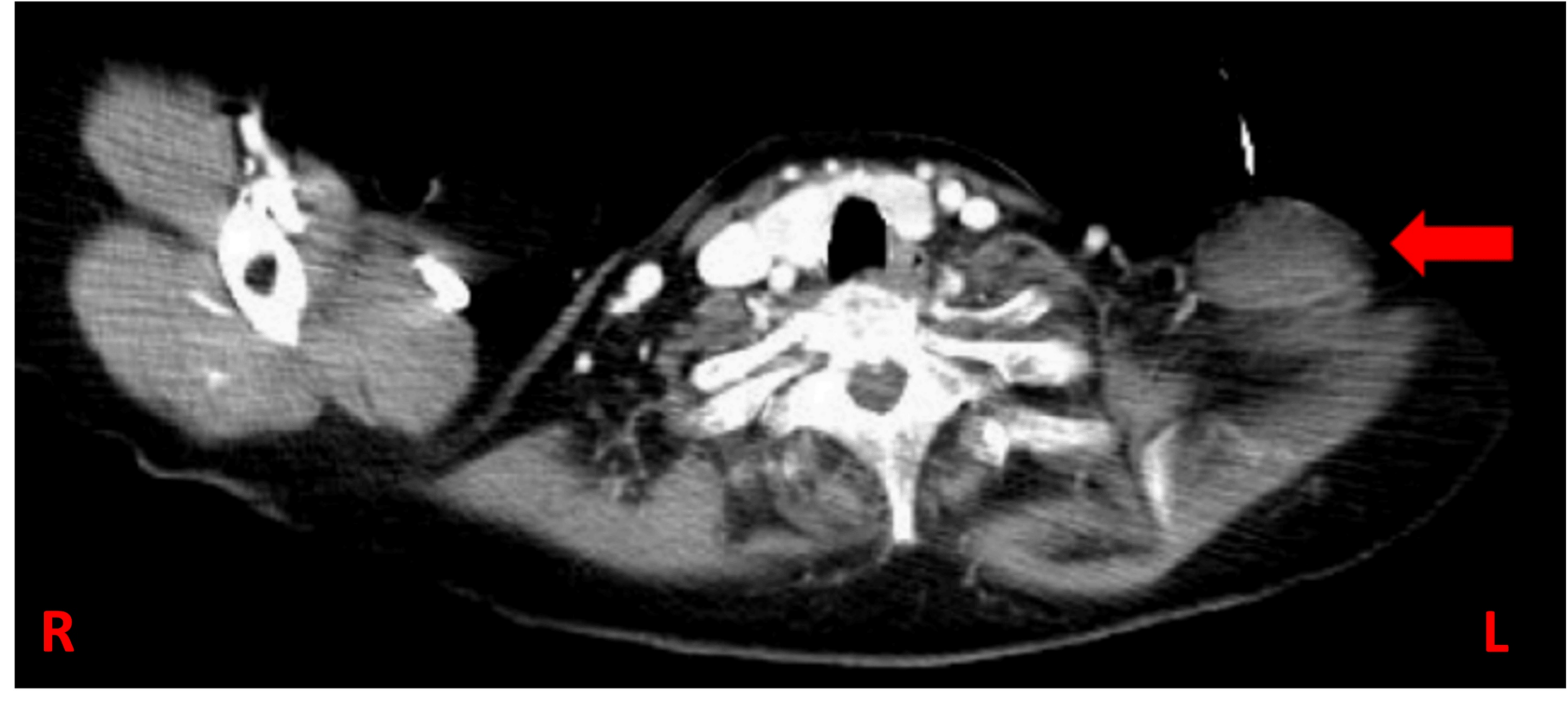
CT scan of the oval SCL lesion in the left supraclavicular region Red arrow: SCL visualized via CT scan SCL: Spindle cell lipoma; L: Patient’s left side; R: Patient’s right side
